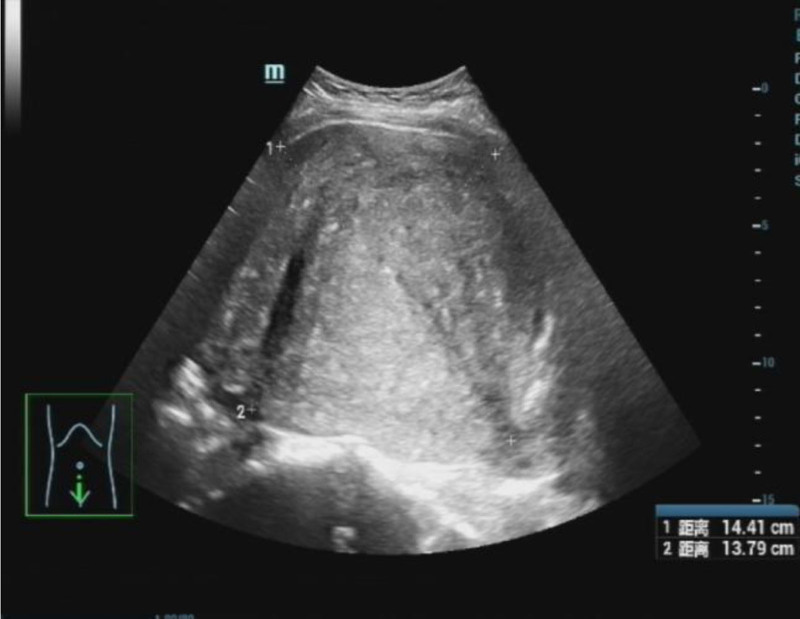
The ultrasound revealed a uterus displaying signs of adenomyosis.
